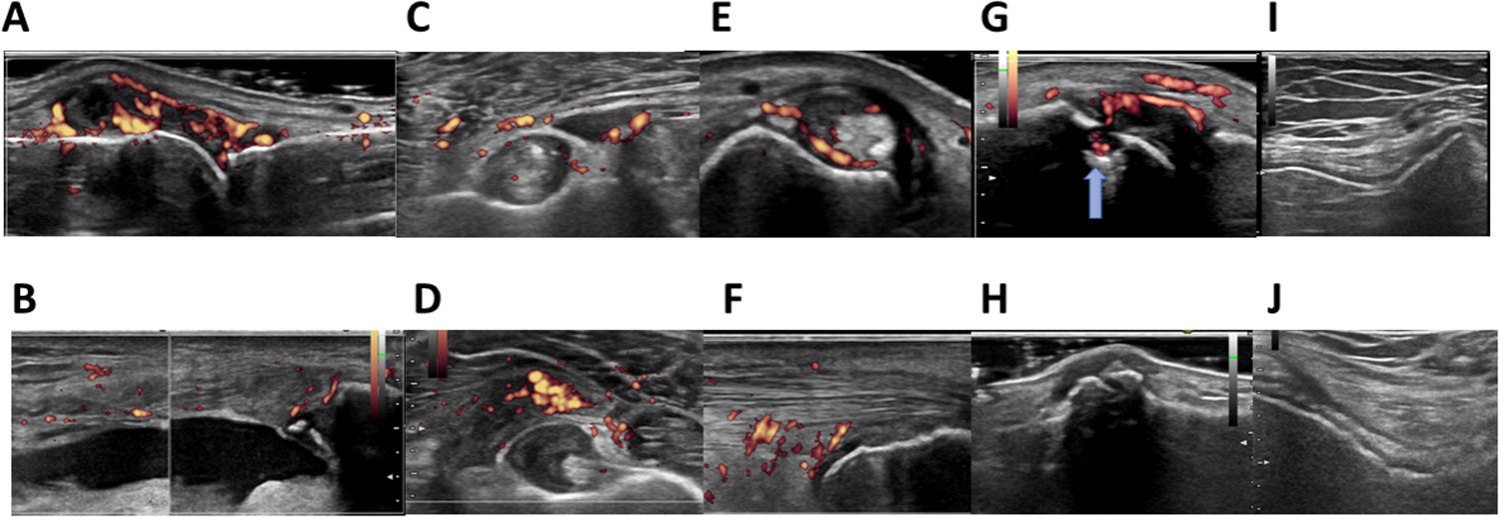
Typical pathological findings of MSUS in inflammatory arthritis. (A) Active synovitis with synovial hypertrophy and power Doppler signals. (B) Synovial fluid retention in the knee joint. (C, D) Bursitis in a patient with PMR and RA. (E) Tenosynovitis in the extensor carpi ulnaris tendon. (F) Achilles tendon enthesitis. (G) Bone erosion in a patient with active RA. (H) Osteophyte on the proximal inter-phalangeal joint in an osteoarthritis patient. (I) Cartilage loss. (J) Crystal deposits on the hyaline cartilage of the knee joint in a patient with calcium pyrophosphate dehydrate deposition disease. MSUS, musculoskeletal ultrasonography; PMR, polymyalgia rheumatica; RA, rheumatoid arthritis.
